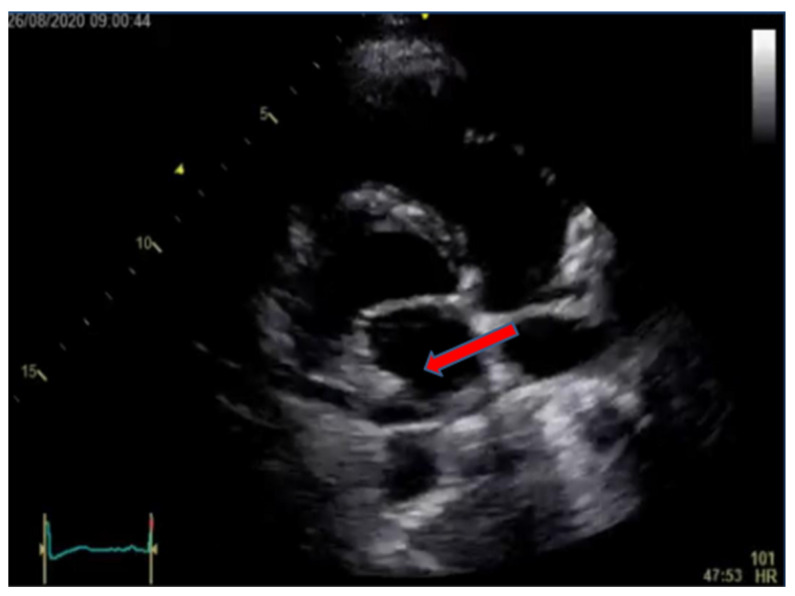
Transesophageal echocardiogram (TEE) showing large tricuspid vegetation on mid-esophageal four-chamber view (arrow showing tricuspid vegetation).
